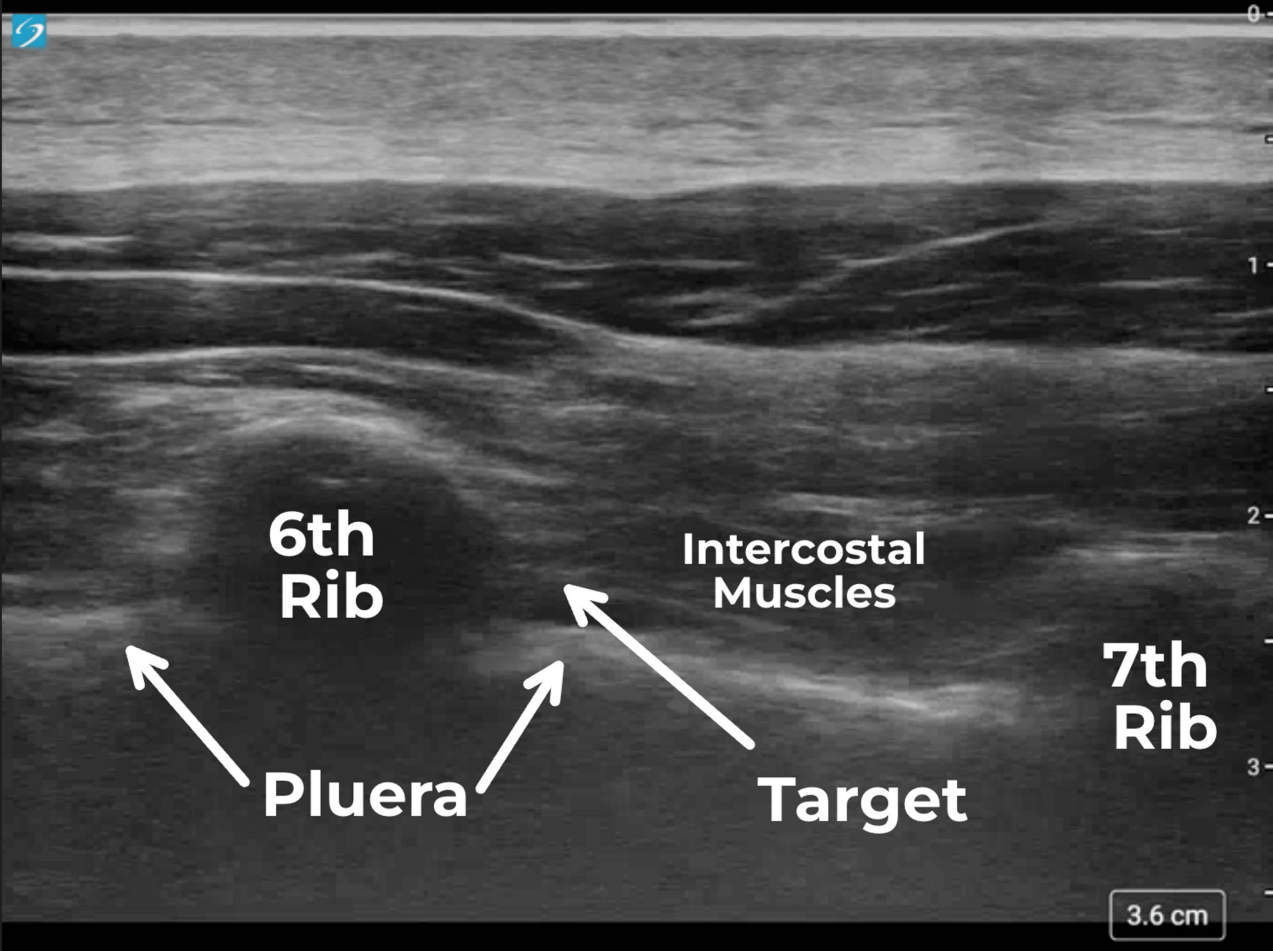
Ultrasound image of the 6th intercostal nerve being targeted for cryoneurolysis.
